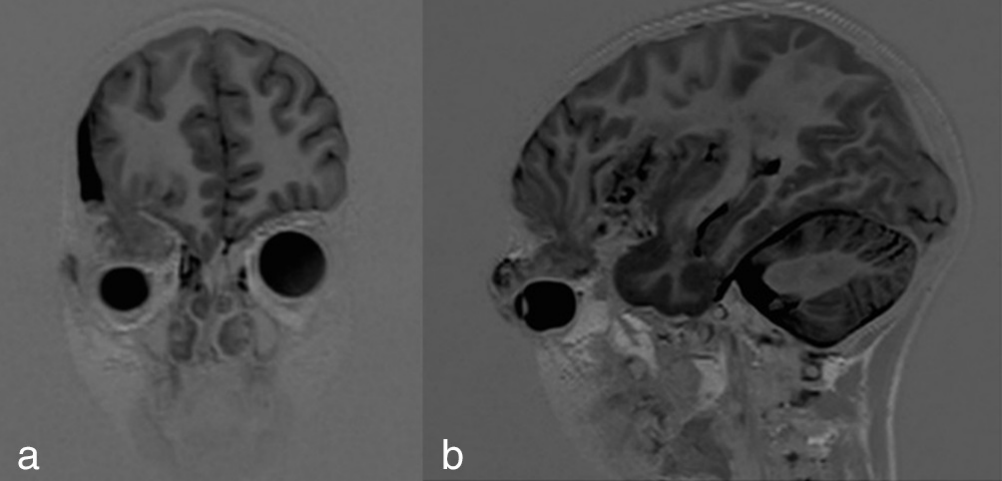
(a, b) Coronal and sagittal thin section *T*_1_ weighted MRI showing herniation of the brain tissue from the cranial cavity into the right orbit.
